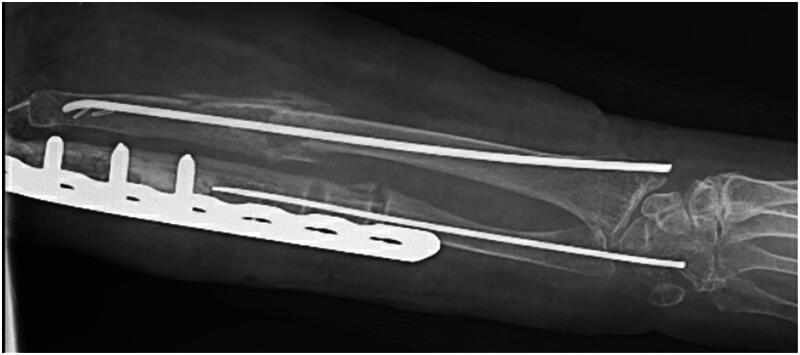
X-Ray 13 days following fracture fixation using TENS showing callus formation.
